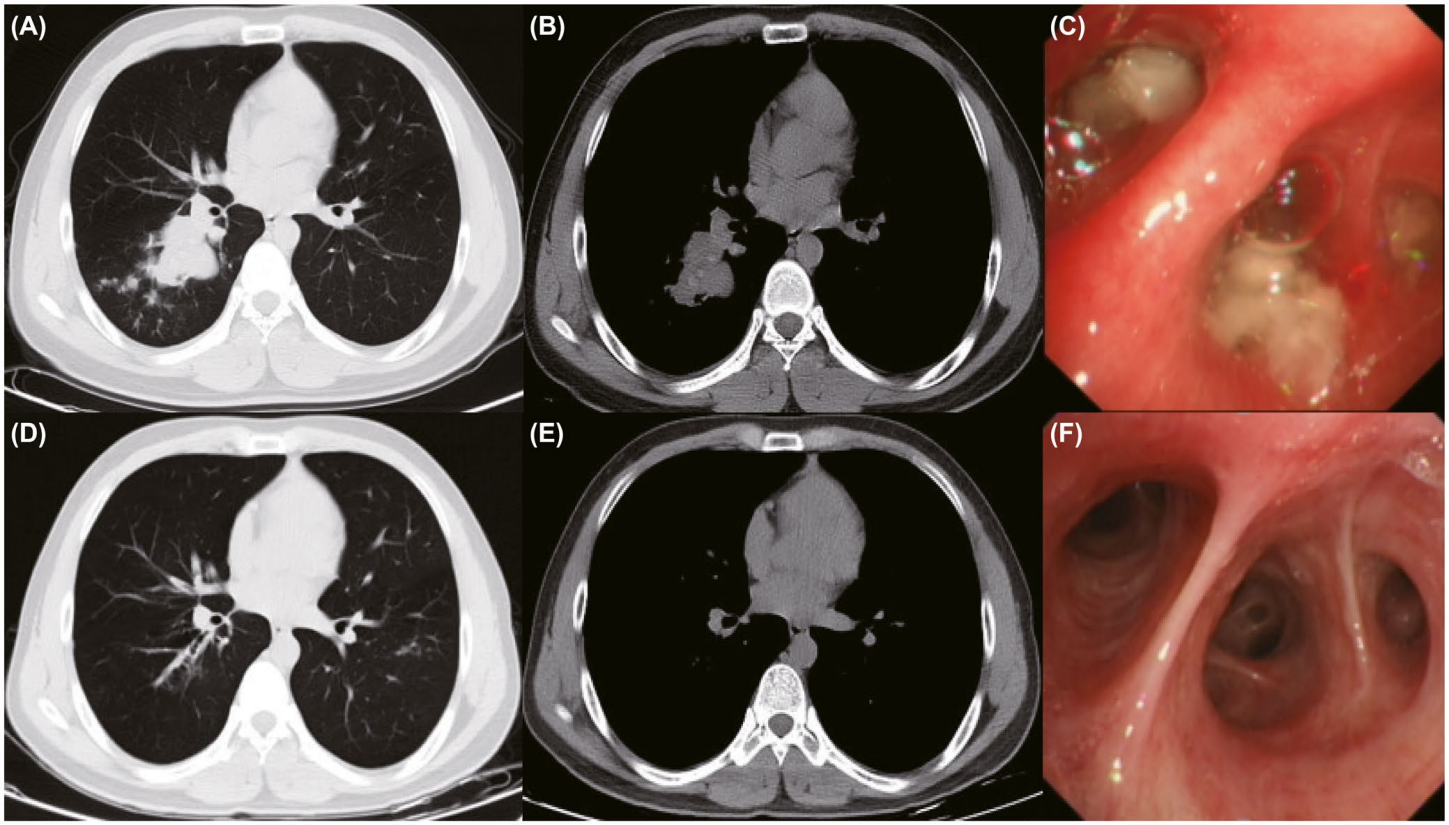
CT scans and bronchoscopy images of LAM before and after the comprehensive treatment. (A,B,C): before the treatment. (D,E,F): after the treatment
